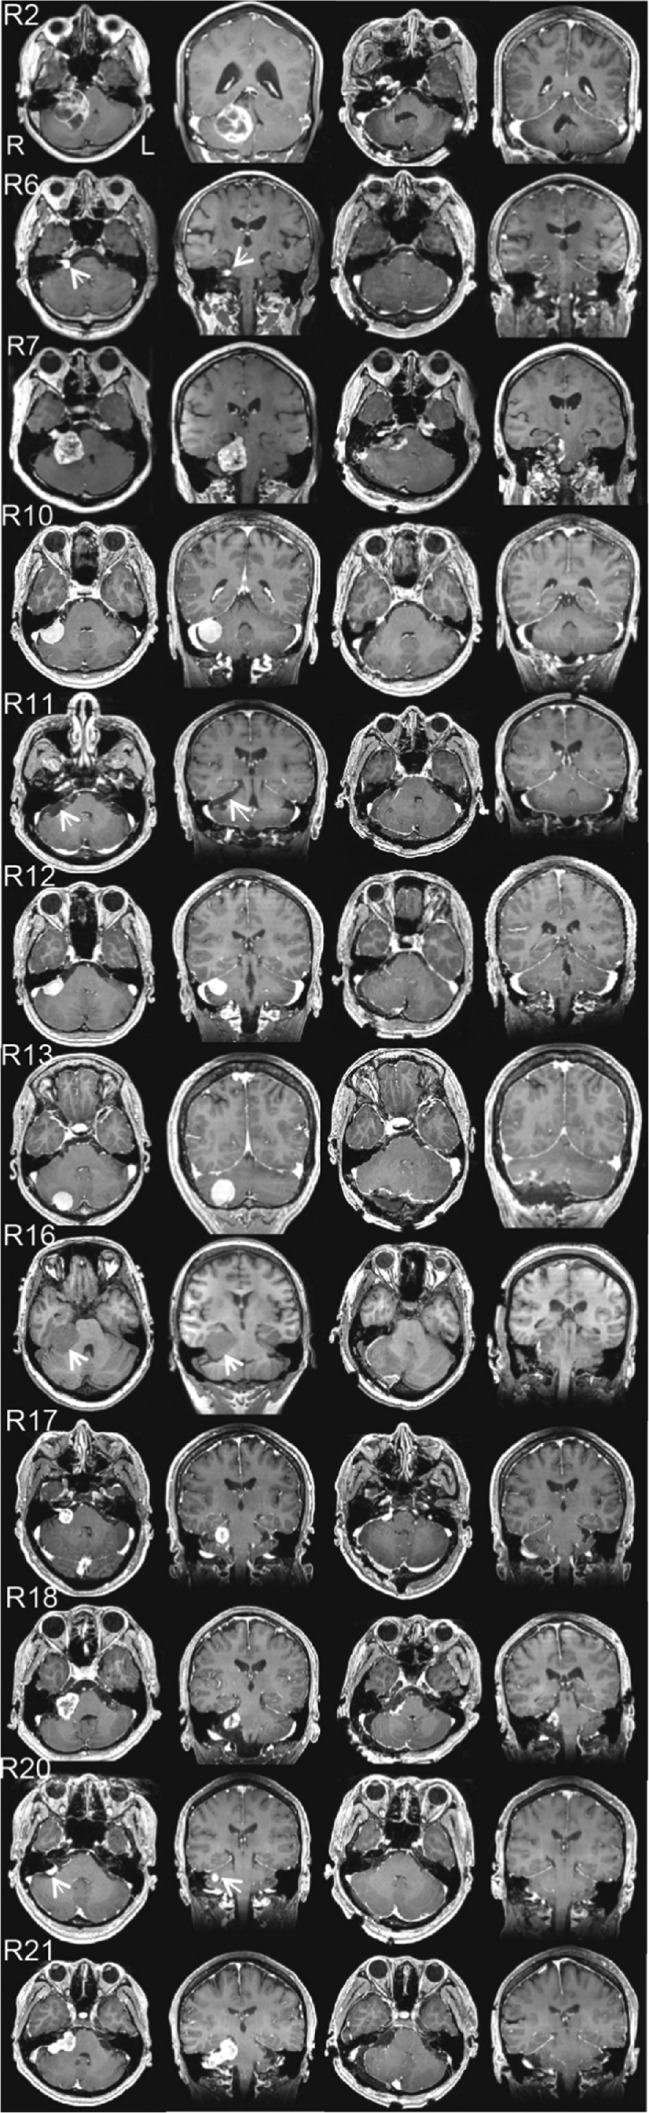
Panels of pre- and postoperative gadolinium-enhanced T1-weighted MRI of cases R2, R6, R7, R10, R11, R12, R13, R16, R17, R18, R20, and R21 (from *top* to *bottom*). *Rows* show axial and coronal images before the operation, and axial and coronal images after the operation (from *left* to *right*). Since Case R16 is subject to asthma, T1-weighted MRI was performed without contrast medium
